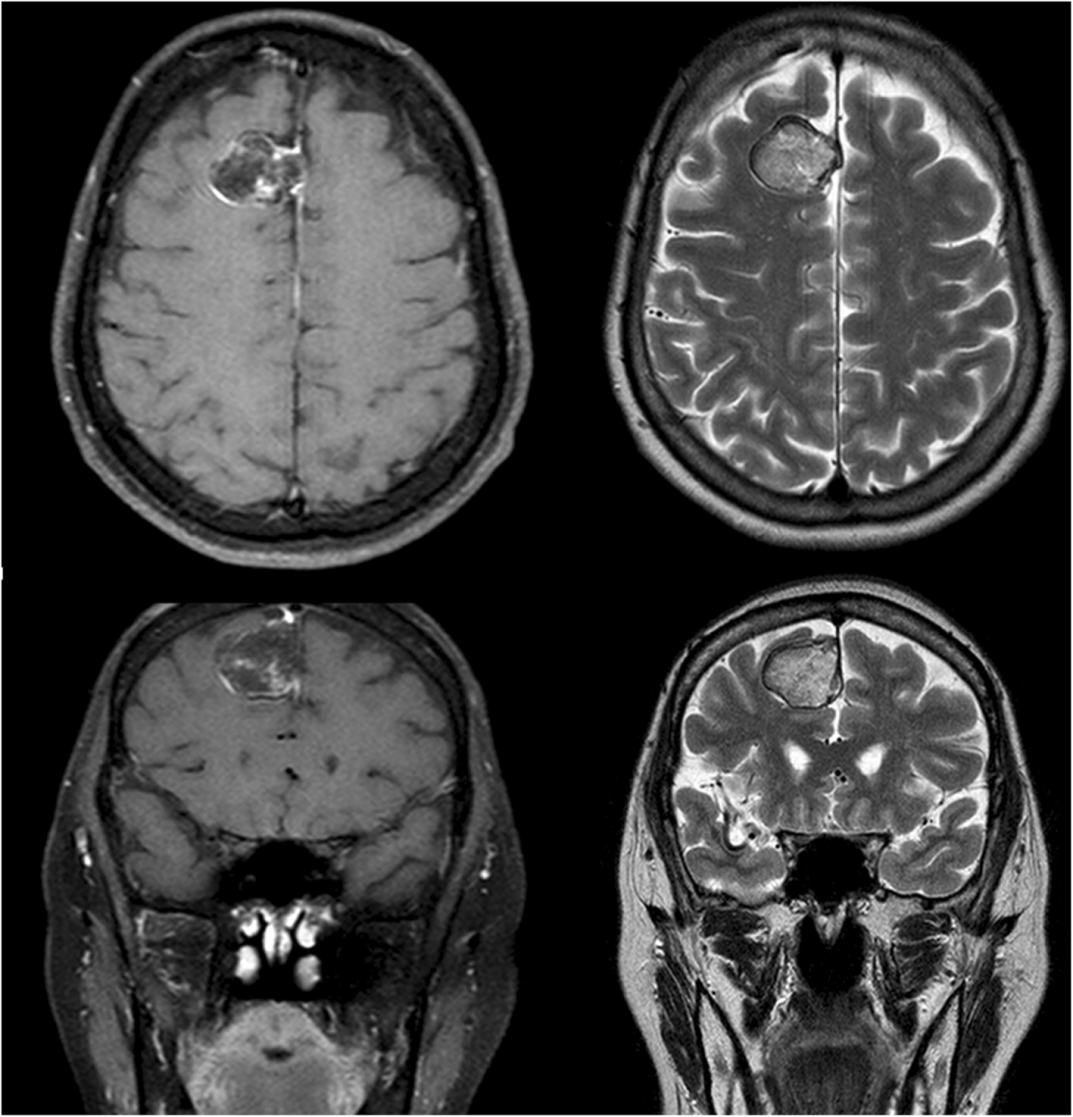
Magnetic resonance imaging (MRI). Axial and coronal contrast-enhanced T1-weighted images with fat saturation show a 2.5-cm falx-based mass with minimal marginal enhancement in the right parasagittal region. The mass is hyperintense with a hypointense rim on T2-weighted images.
